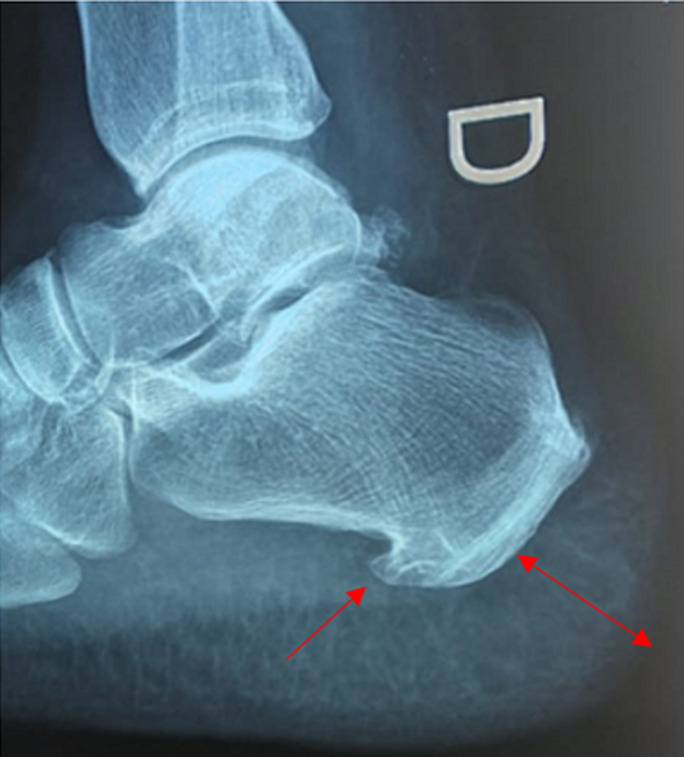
X-ray of foot showing the plantar pad and the osteophyte
